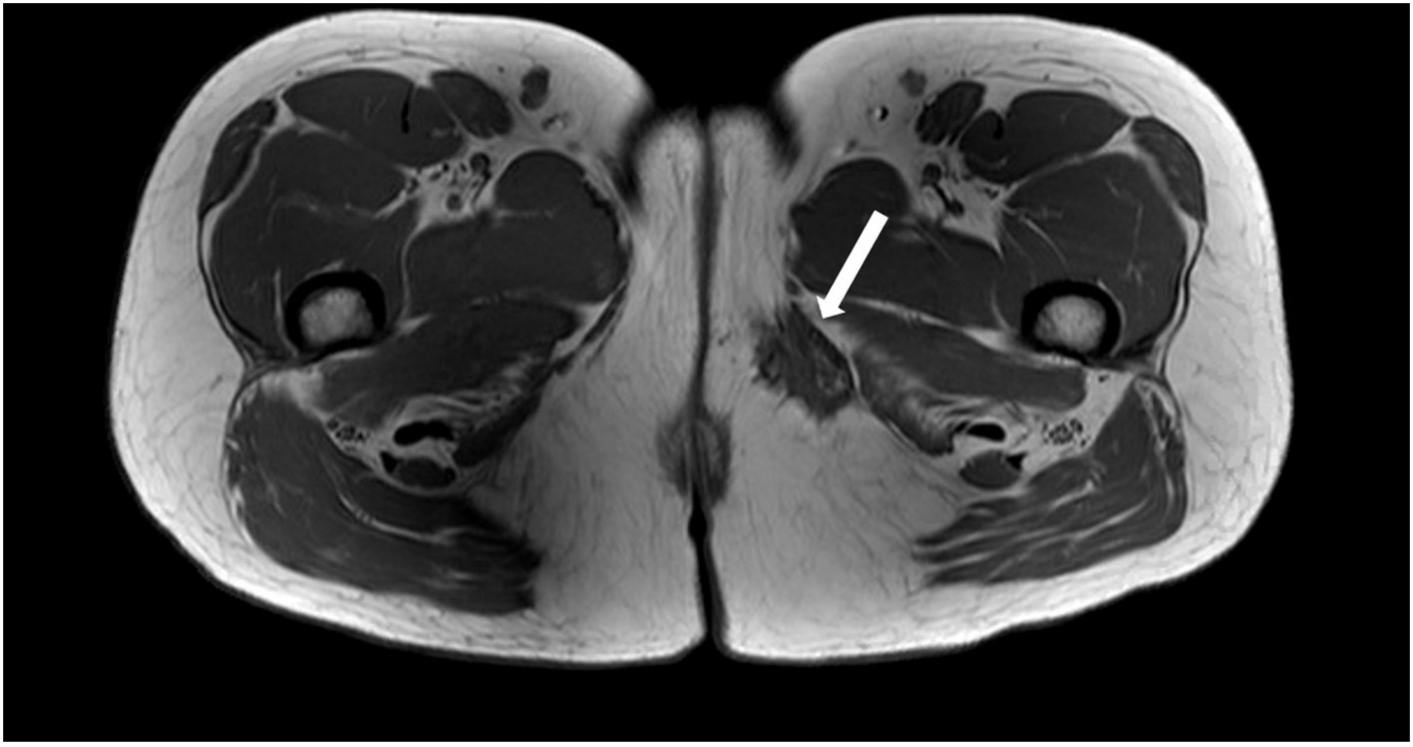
Axial T1 weighted MRI image demonstrating a spiculated hypointense lesion of the left medial thigh.
